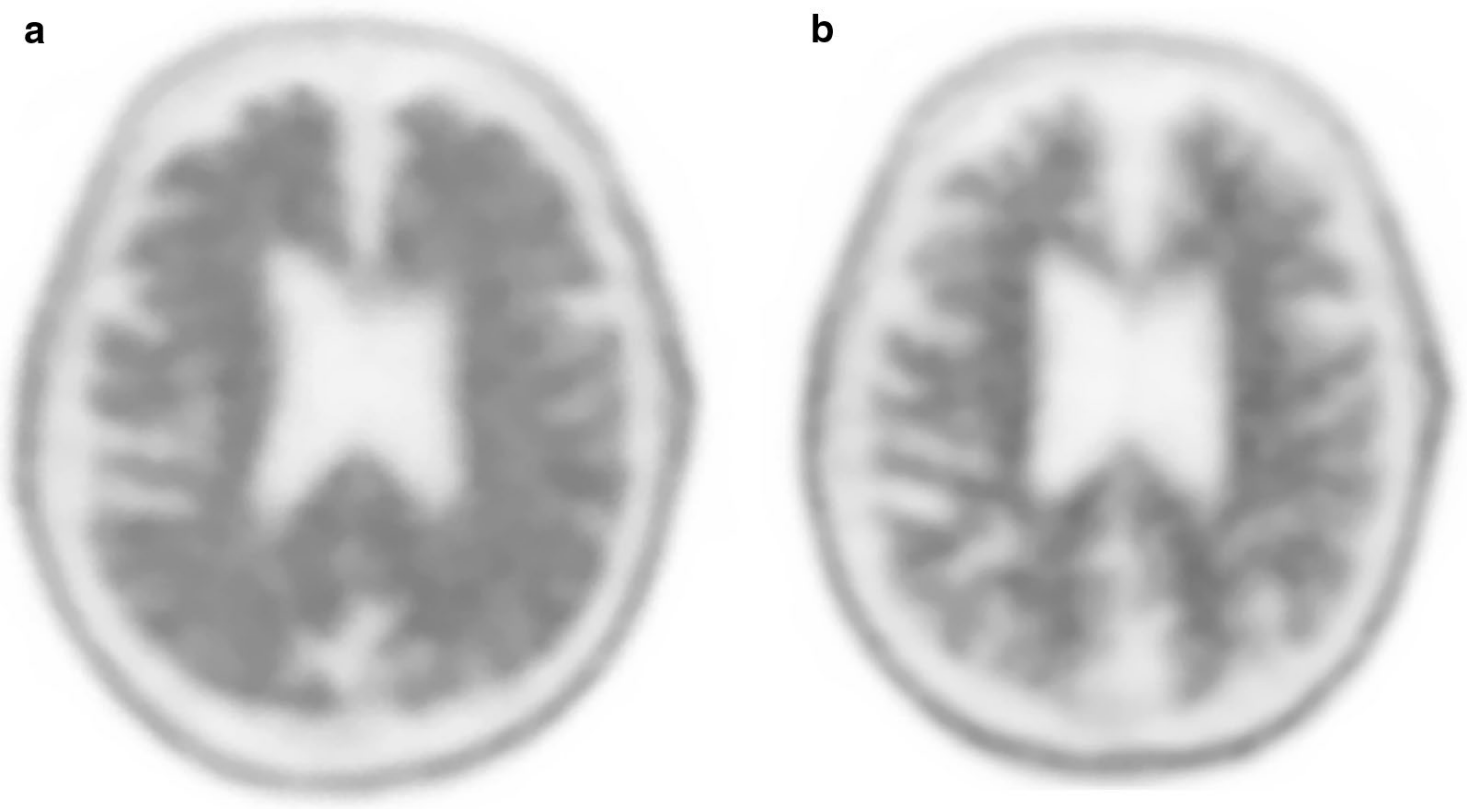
A 83-year-old male with typical AD on anti-Aβ monoclonal antibody clinical trial drug. Pre-treatment florbetapir PET/CT demonstrates substantial cortical amyloid burden with kissing hemispheres in midline (a). Following treatment there is significant reduction in amyloid uptake with visualization of corticomedullary differentiation reminiscent of a normal scan (b)
